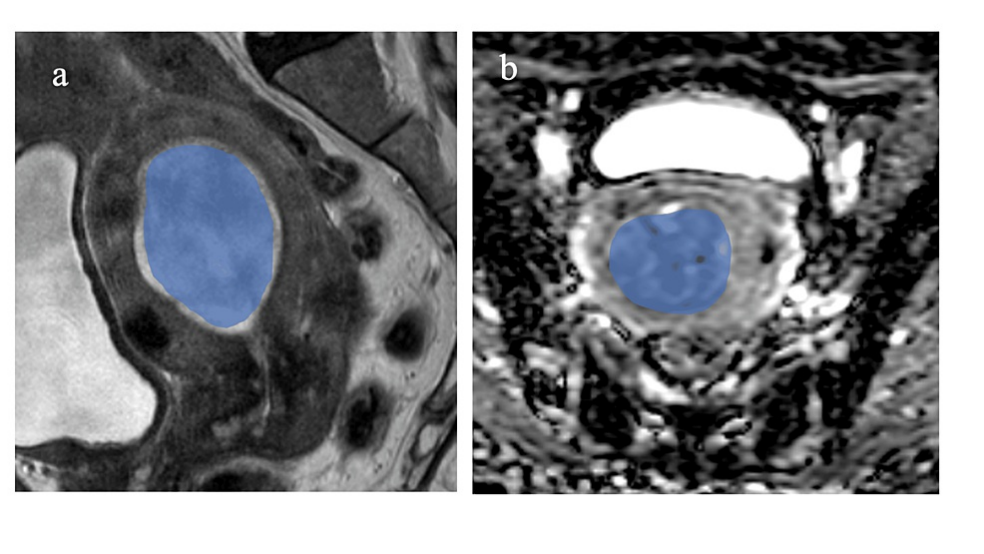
Segmentation in a 75-year-old woman with uterine carcinosarcoma (a) Sagittal T2-weighted image and (b) axial apparent diffusion coefficient map. The maximum tumor slice of each sagittal or axial T2-weighted image and axial apparent diffusion coefficient map is selected. An irregular two-dimensional region of interest is drawn manually to contain the outline borders of the entire region of the largest cross-sectional tumors using open-source software (ITK-SNAP version 3.8.0).
